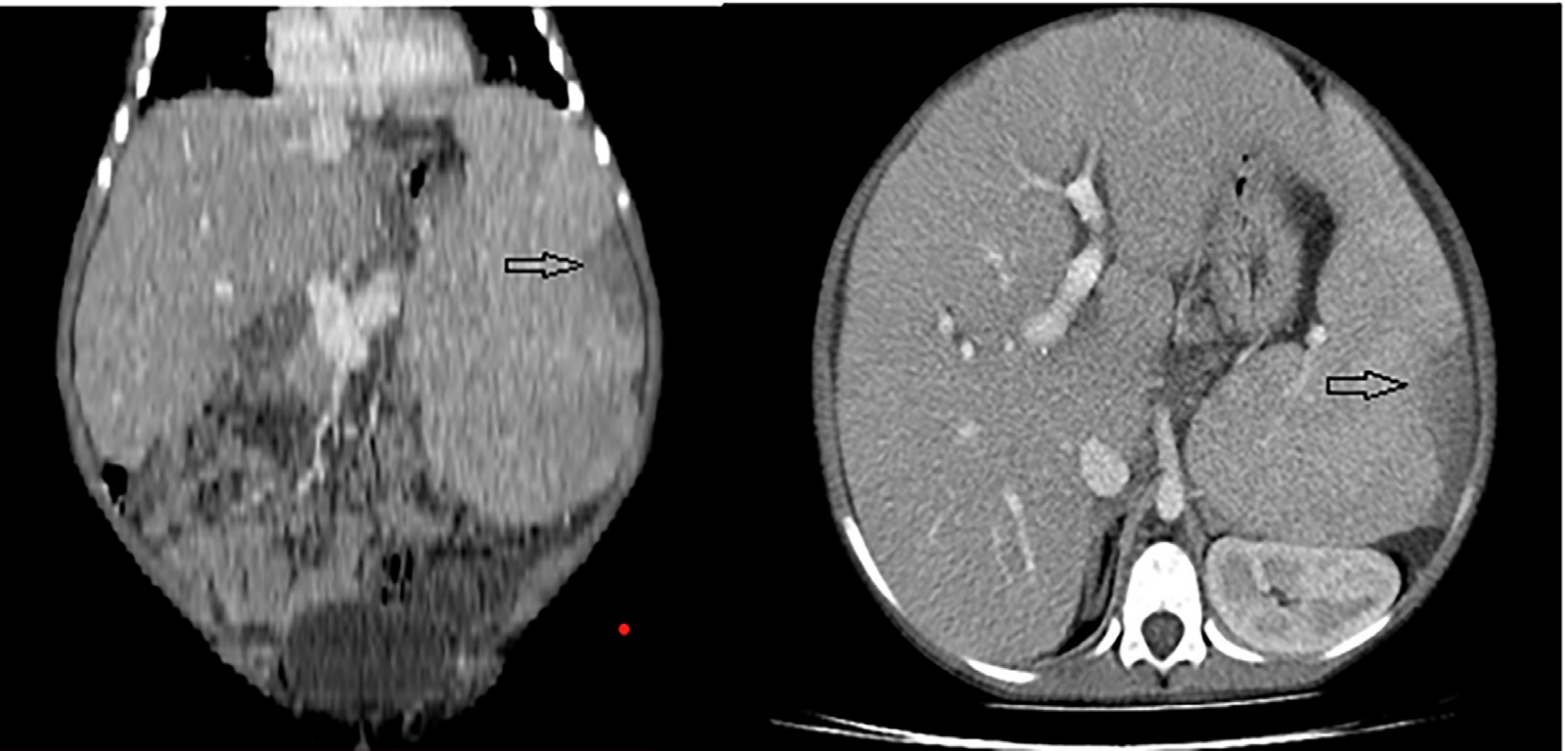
Hepatosplenomegaly and splenic infarct. Coronal and axial postcontrast CT scan of the abdomen showing hepatosplenomegaly and wedge-shaped splenic infarct (Arrow).
